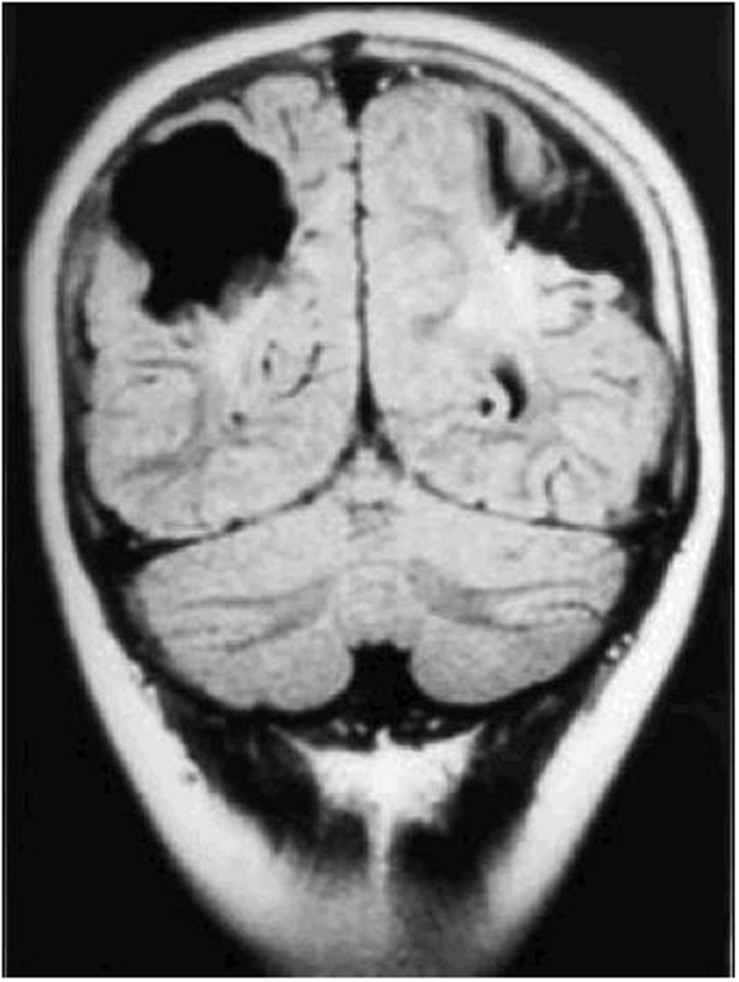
Coronal CT scan of bilateral posterior parietal lobe scarring in a 10 years old boy with features of Balint syndrome. (Reproduced by the author GN Dutton, from Gillen and Dutton, 2003.)
